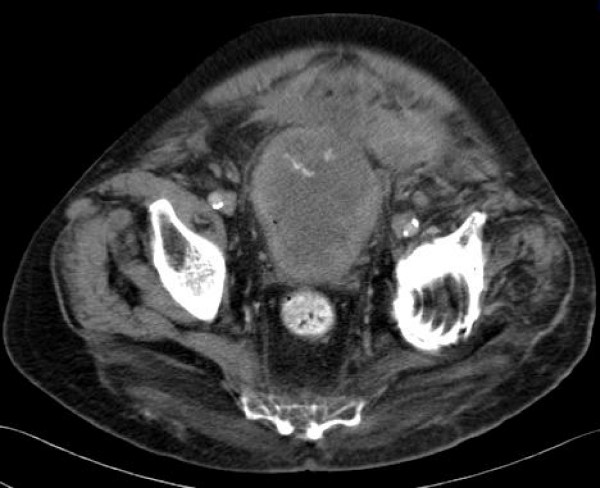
Computed tomography scan seven months afterdiagnosis shows extravesical tumour extension and bowel invasion.
